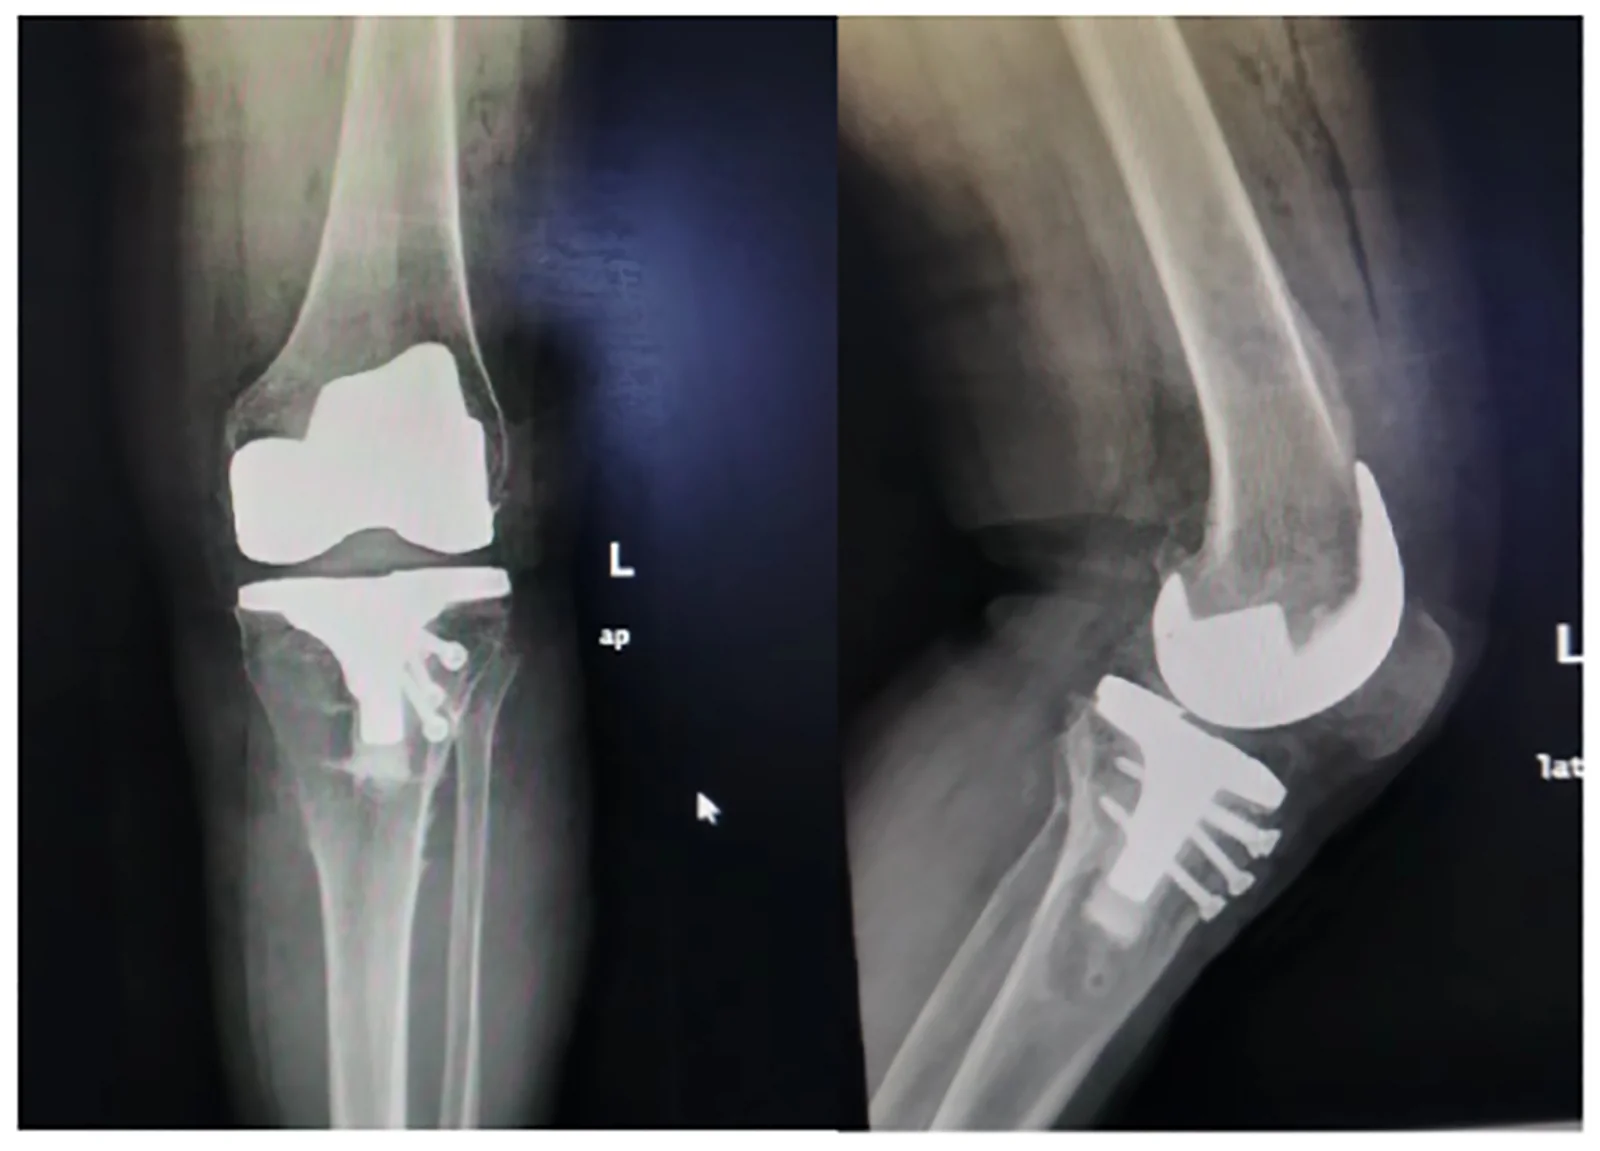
Postoperative anteroposterior and lateral view radiographs of the left knee.
